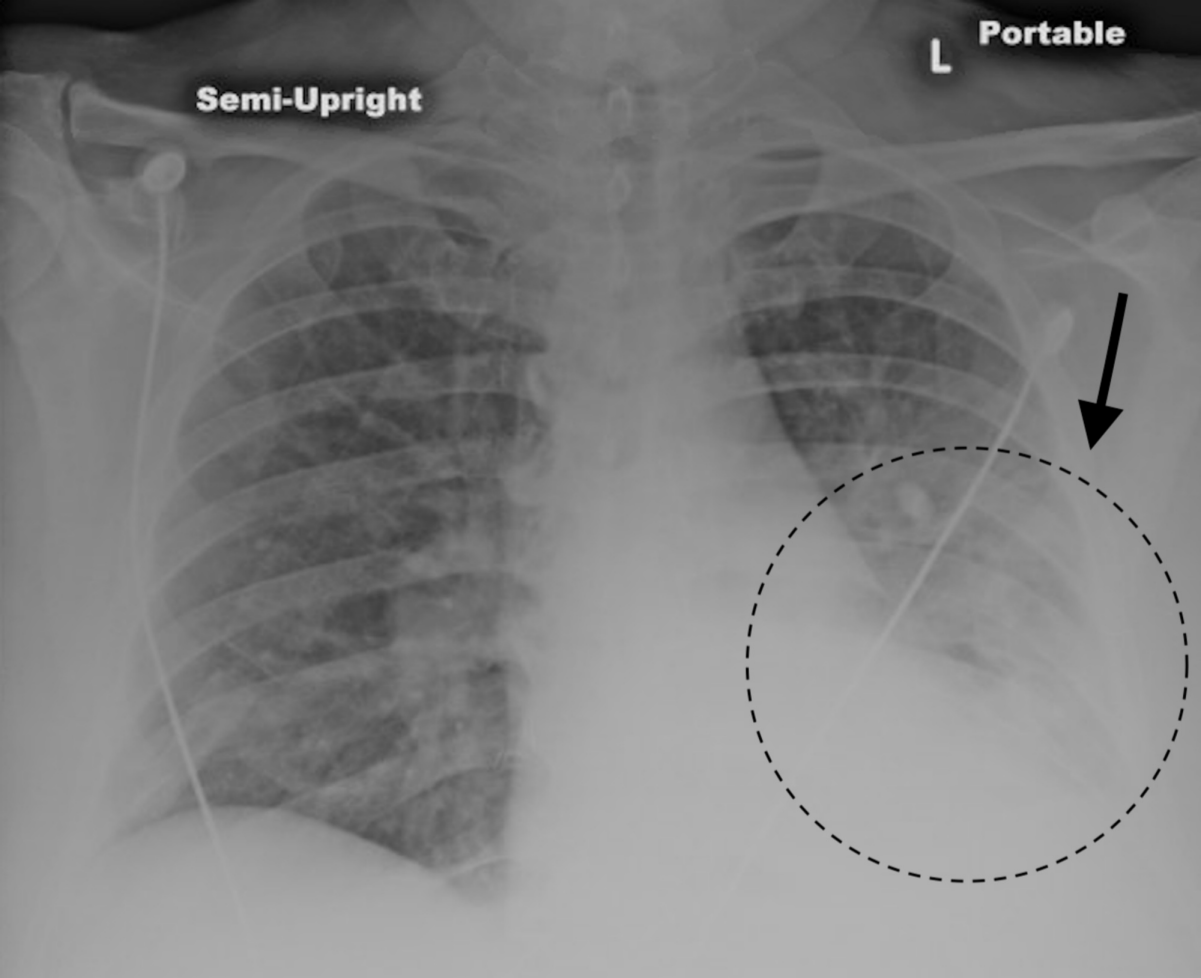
Chest x-ray on admission demonstrating a left lower lobe opacity.
